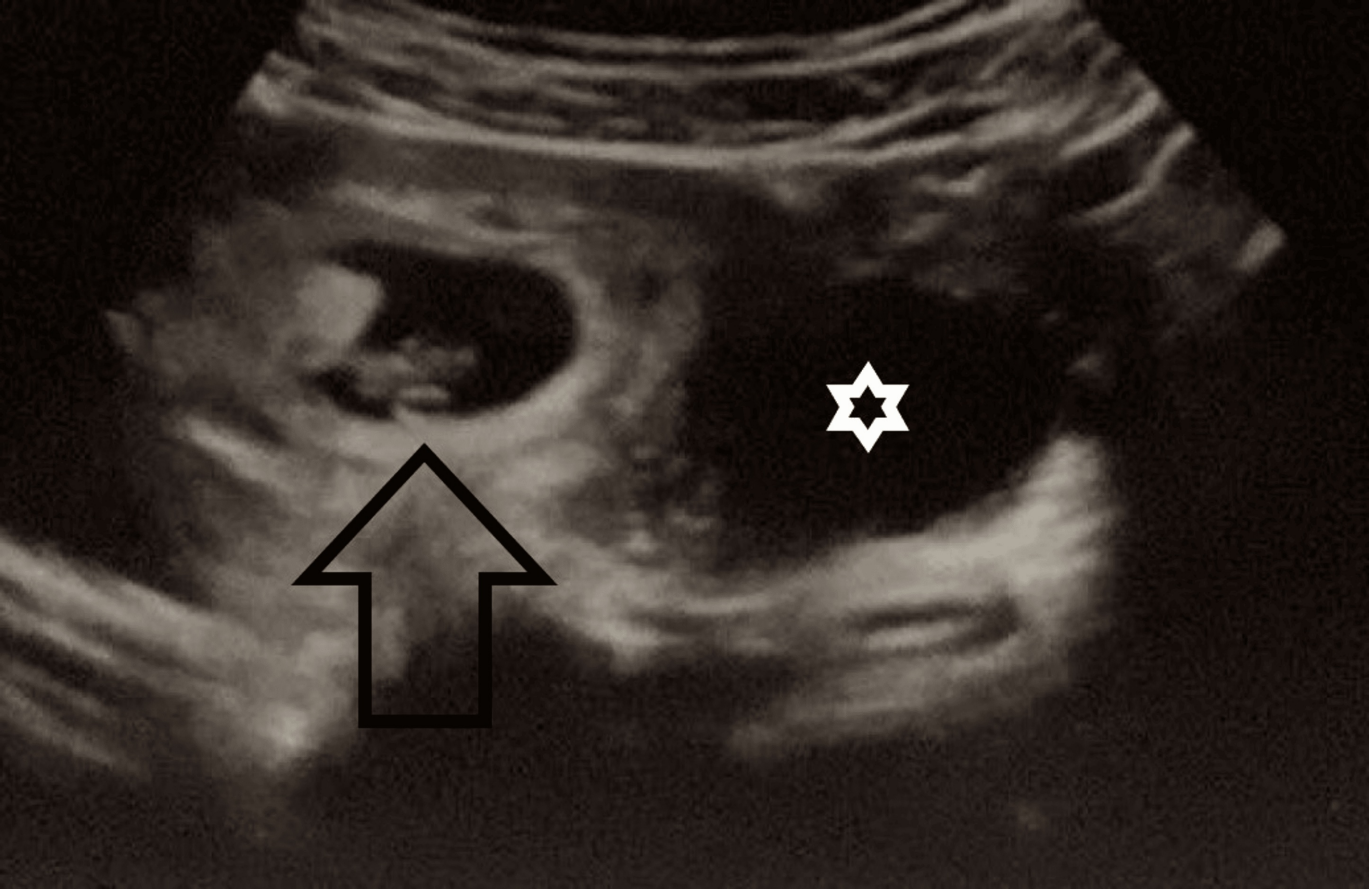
Ultrasound showing a normal developing intrauterine pregnancy with free-floating abdominal fluid in the Douglas pouch and lower abdomen. The black arrow points to the normal gestation sac in the uterine cavity. The white star presents the free-floating fluid in the Douglas pouch.
